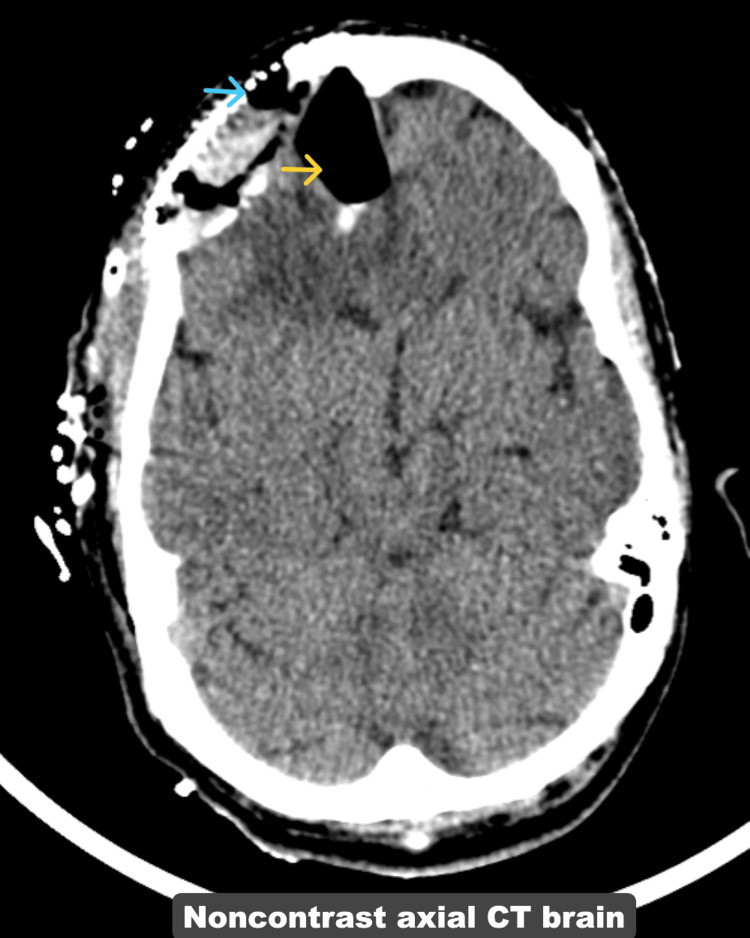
Day 41 postoperative non-contrast axial CT brain showing reduction in the pneumatocele and midline shift The yellow arrow shows a significant reduction in the previously noted intracranial air collection (intracerebral pneumatocele) in the right basifrontal region. The blue arrow shows postoperative changes in the right frontal calvaria.
